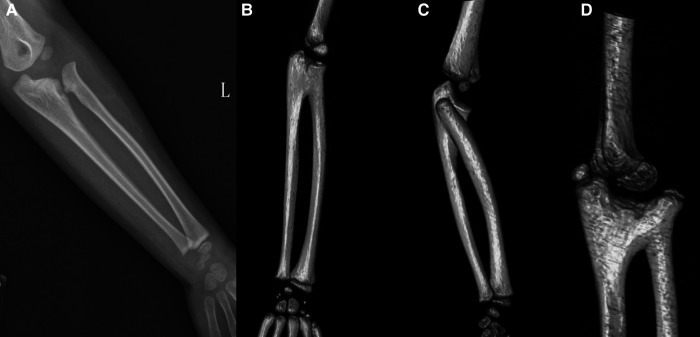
X-ray or CT reconstruction of forearm deformities. (A) C & O Type I; CMPOS Type I. (B) C & O Type II; CMPOS Type II. (C) C & O Type III; CMPOS Type III. (D) C & O Type IV; CMPOS Type II.
